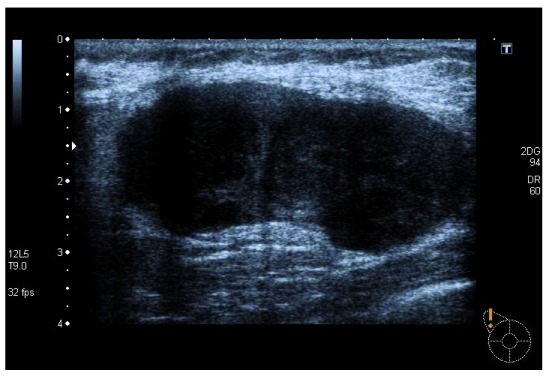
Ultrasonography of nodular formation at the upper external quadrant of the right breast.
